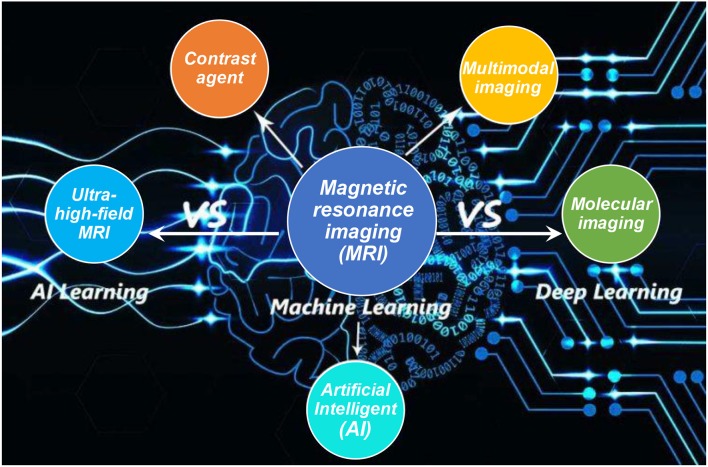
MRI and AI to varies of imaging development.
